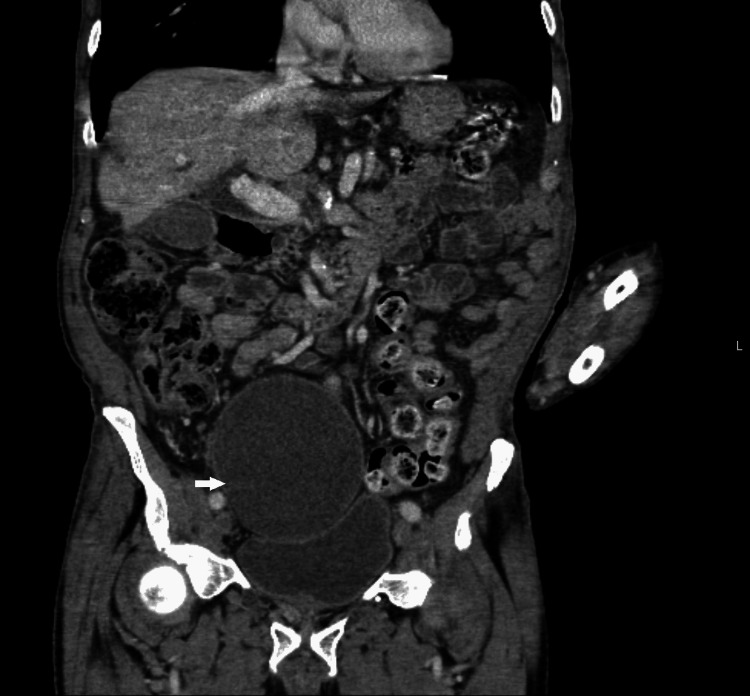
CT Abdomen (Coronal View): Large simple cyst originating from the right seminal vesicle and compressing the anterior bladder wall
